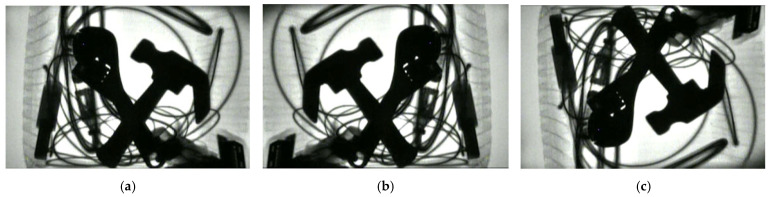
Image flipping of the X-ray image of prohibited items. (a) The original X-ray images; (b) horizontal flipping; (c) vertical flipping.
